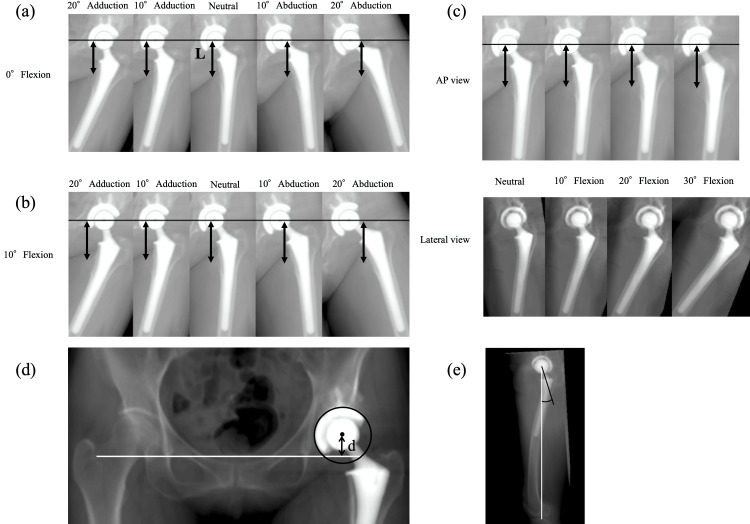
Image analysis (a-c) Digitally reconstructed radiography images were reconstructed in various limb positions. The vertical distance from the X-axis (the solid black line) to the most prominent point of the lesser trochanter (the black arrow, L) was measured on each image. (d) The vertical distance (the black arrow, d) from the epiphyseal head center (the solid black circle) to the trans-teardrop line (the solid white line) was used for leg length measurement. (e) On the digitally reconstructed sagittal image, the torsion angle was measured of the femoral neck axis (the solid black line) relative to the femoral mechanical axis (the white solid line).
